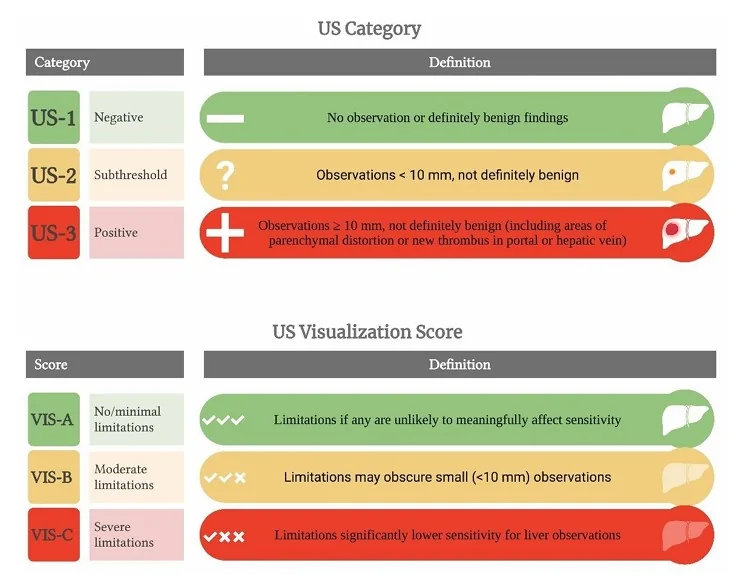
Summary of the LI-RADS^®^ Ultrasound Surveillance (Version 2024).
